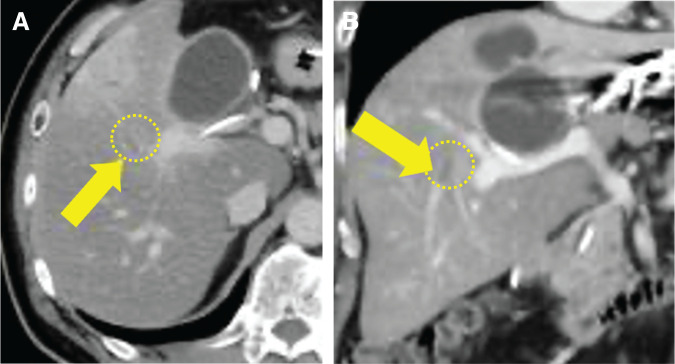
Enhanced CT after thrombus formation. (A, B) The arrows indicate the RHV, and the area enclosed by the broken line represents a blood clot. RHV, right hepatic vein
